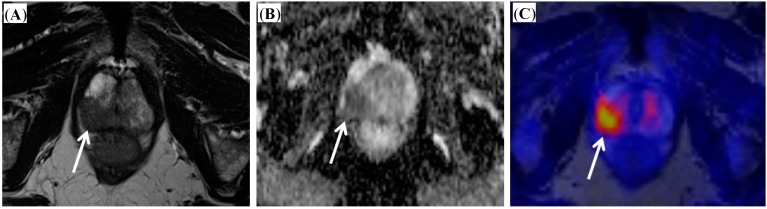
Patient with biopsy proven prostate carcinoma (Gleason score 4 + 3 = 7). (A) T2-weighted image presenting a hypo-intense lesion of the right peripheral zone; (B) ADC map indicating restricted diffusion within the tumor; (C) Fused PET/MR image depicting pathologically increased choline uptake of the tumor. (Arrows indicate prostate carcinoma).
